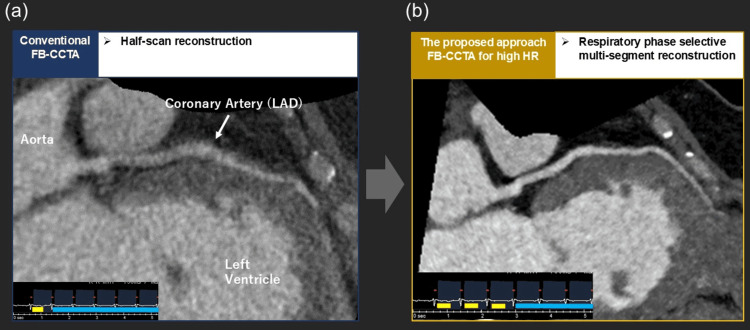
Comparison of coronary image quality between conventional and respiratory phase-selective reconstruction Representative coronary CT angiography images reconstructed using (a) conventional half-scan reconstruction under free-breathing conditions and (b) respiratory phase-selective multi-segment reconstruction based on TDC-derived respiratory phase information. Respiratory phase–selective reconstruction demonstrates reduced motion artifacts and improved coronary delineation. FB-CCTA: Free-breathing coronary CT angiography, TDC: Time-density curve, HR: Heart rate, LAD: Left anterior descending artery
